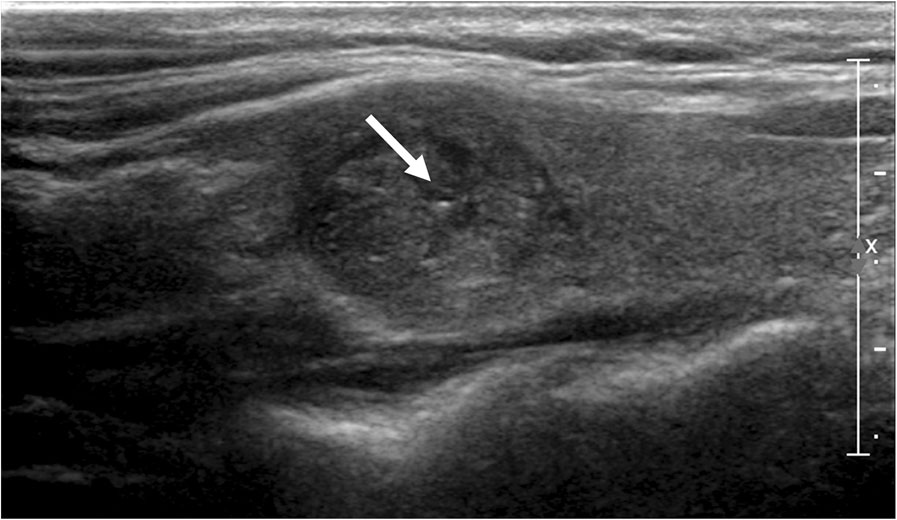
Echogenic foci associated with malignancy. 36-year-old female with thyroid nodule. Ultrasound image shows a 1.8 cm hypoechoic, solid nodule. Echogenic foci with no posterior acoustic artifact (Type 1, arrow). Biopsy result was papillary carcinoma and was confirmed at surgery
